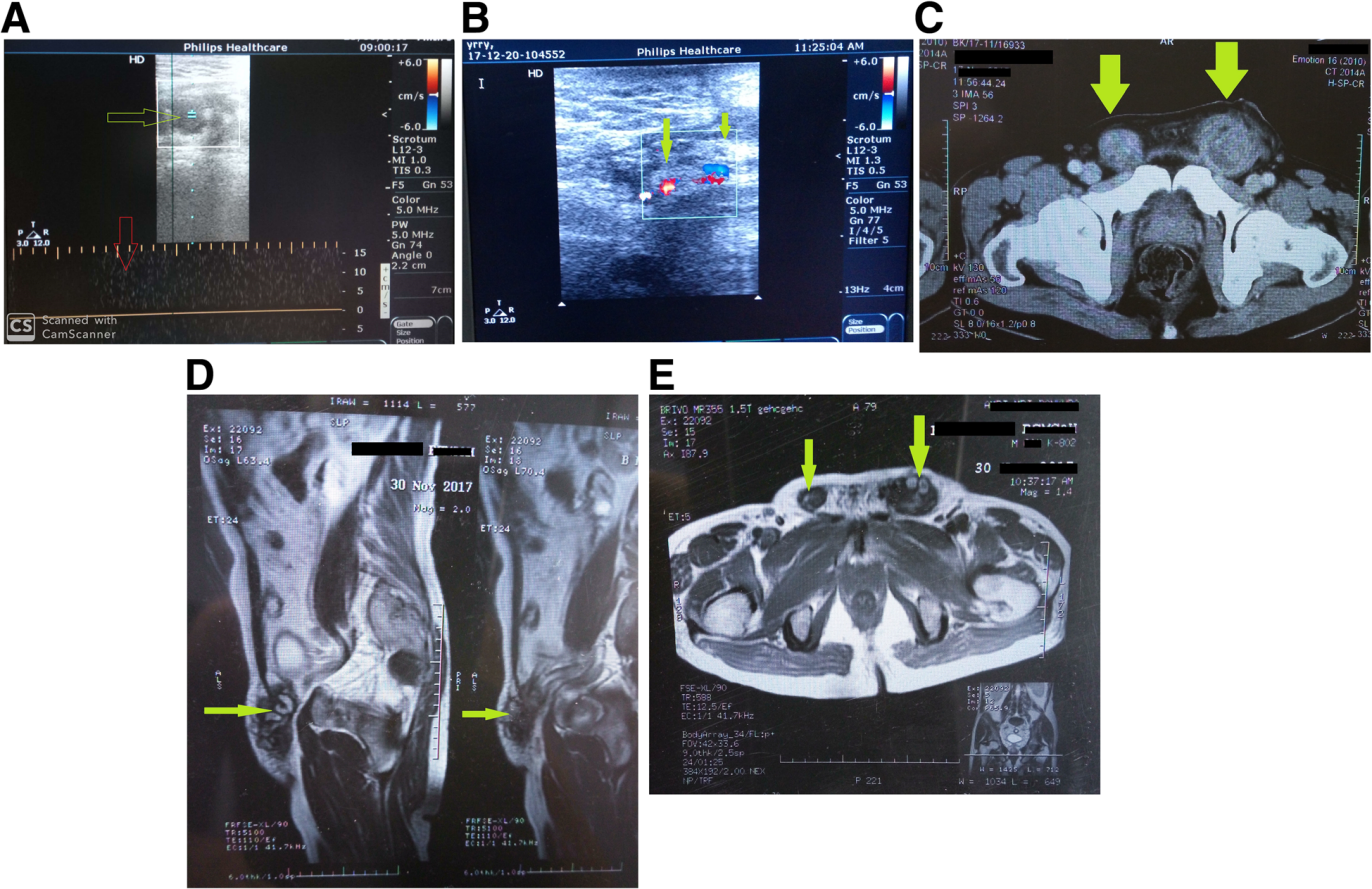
a Grayscale ultrasonography demonstrate dilated, non-compressible, thrombosed tubular venous structure with increased wall thickness within the left spermatic cord. Within this tubular structure, focal echoes that belong to thrombus (green arrow) can be seen, but no vascular flow curve can be seen (red arrow). b On color Doppler ultrasound, no filling with the color was seen in the lumen of this vein within the left spermatic cord. On Doppler ultrasound, filling was seen within the neighboring arterial structure (green arrows), but not within the vein. c Computed tomography scan showing grossly distended and thrombosed spermatic veins (green arrows). d Sagittal section and e transverse section: fat-compressed axial T1 magnetic resonance images demonstrated thrombosed tubular venous structure (green arrows) with increased wall thickness and focal diameter increase within the bilateral spermatic cord. Within this venous structure, intraluminal signal intensity was increased
